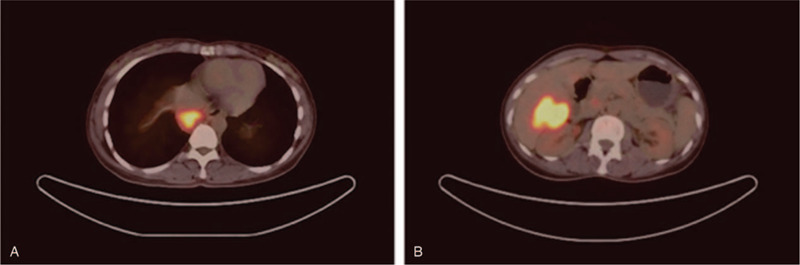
Positron emission tomography/computed tomography findings. A, The imaging revealed a 2.3-cm hypodense lesion in the posterior mediastinum, which shows significantly different levels of FDG uptake (SUV maxy ≈ 8.1). B, The imaging revealed a 6.7-cm hypodense lesion in the S6 lobe of the liver with remarkable FDG uptake (SUV max ≈ 8.1).
